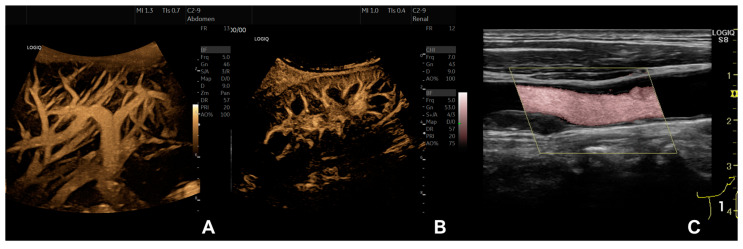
Examples of B-Flow ultrasound imaging of the liver (A), kidney (B), and carotid artery (C). Used with permission of GE Healthcare.
